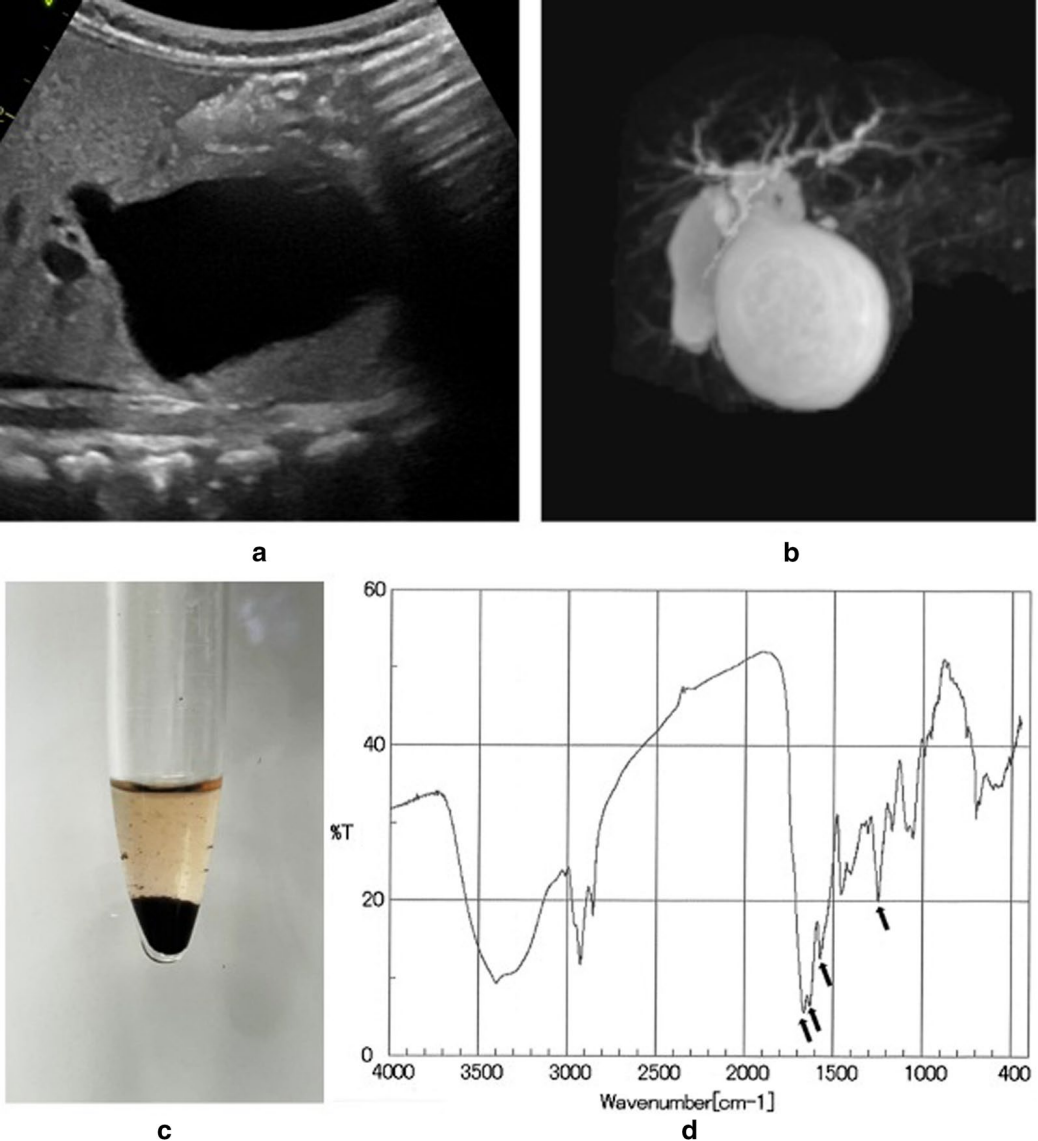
Data of Case 2. a Ultrasonography detected debris in the cyst. b Magnetic resonance imaging showed a cyst of the common bile duct. c The sludge in the bile duct consisted of fine black particles. d Infrared absorption spectra of the debris. Four major absorption bands (arrows) were characteristic of calcium bilirubinate
